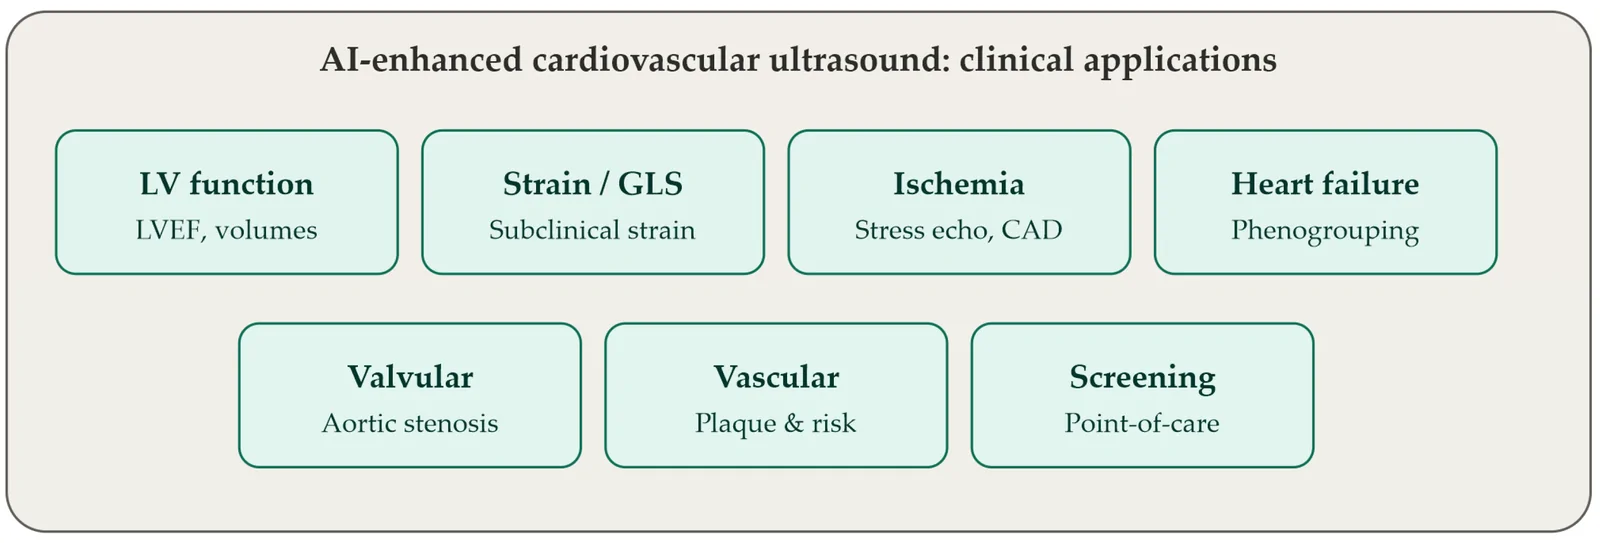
Principal clinical applications of AI-enhanced cardiovascular ultrasound.
